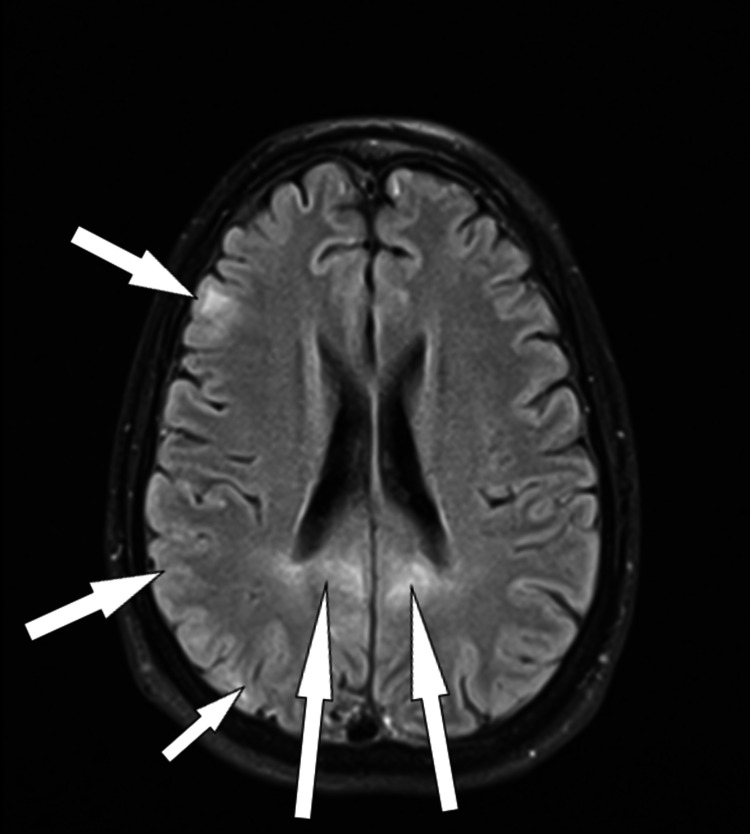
Axial view of FLAIR MRI The arrows signal parasagittal gyriform hyperintensity with bilateral distribution, consistent with PRES FLAIR: fluid-attenuated inversion recovery; MRI: magnetic resonance imaging; PRES: posterior reversible encephalopathy syndrome
